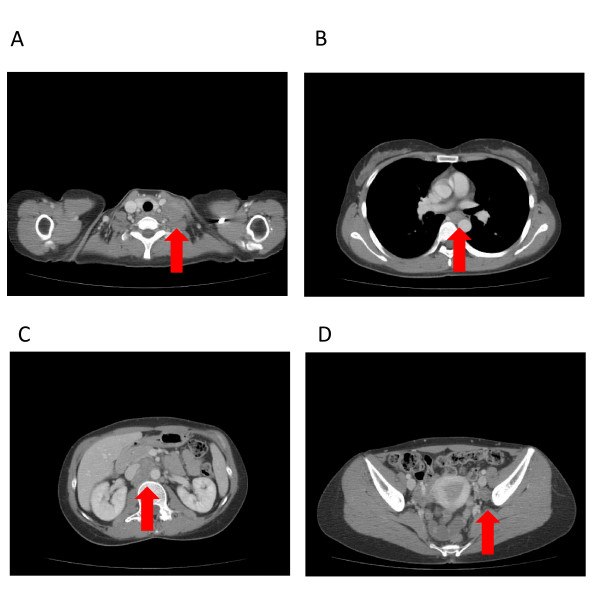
Whole-body computed tomography (CT) findings. Systemic lymph node swelling was observed. (A) Left supraclavicular lymph node metastasis measuring 33 mm in diameter (arrowhead). (B) Middle thoracic para-oesophageal lymph node metastasis measuring 24 mm in diameter (arrowhead). (C) Multiple para-aortic lymph node metastases in the abdomen, the largest one measuring 43 mm in diameter (arrowhead). (D) Left iliac internal lymph node metastasis measuring 18 mm.
